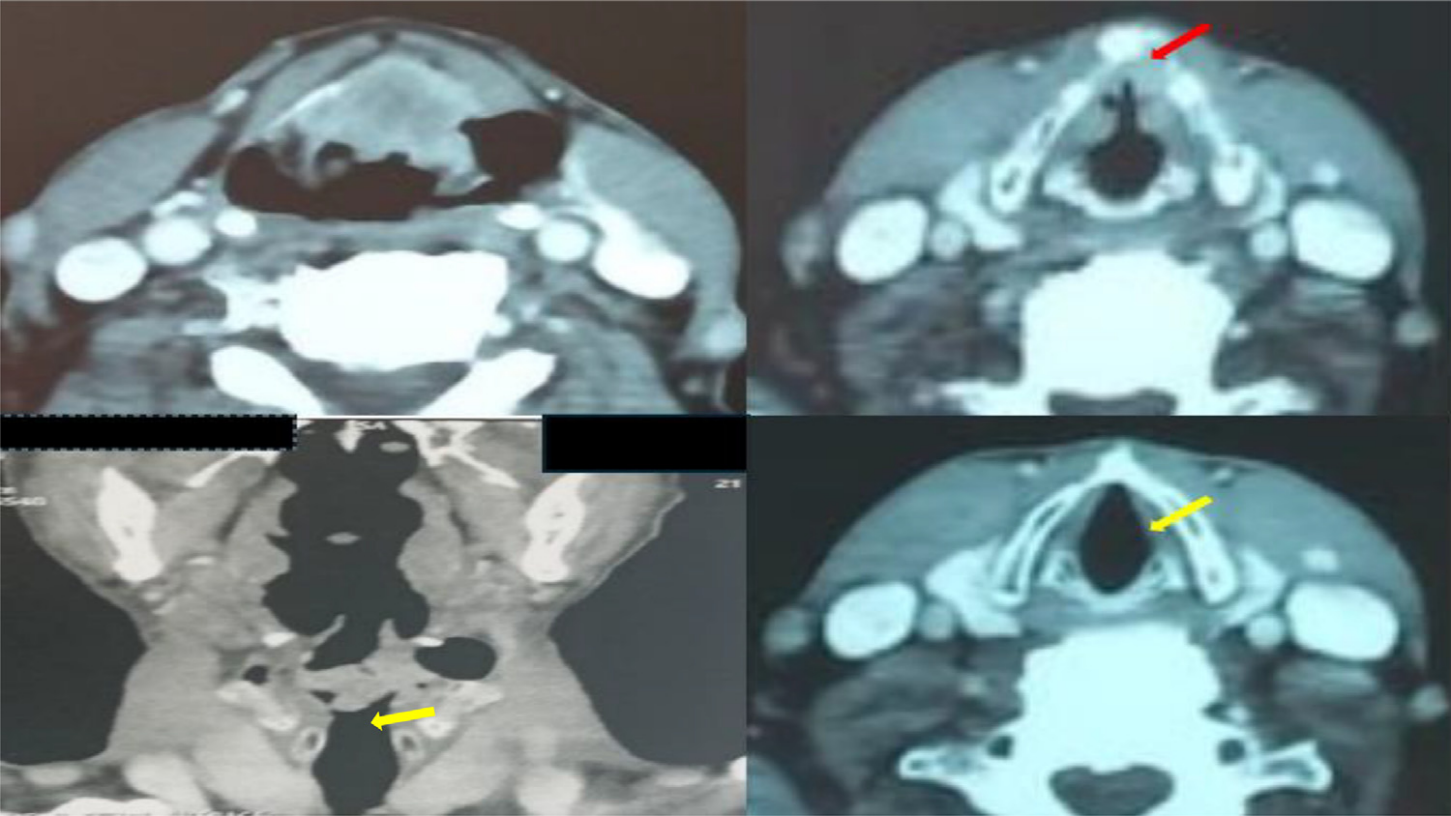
Imaging CT in axial and coronal coupe, showing an irregular process in the glottic and supra-glottic level, reaching the anterior commissure (red arrow). There is no extension to the subglottic area (yellow arrow) or cervical lymph nodes.
